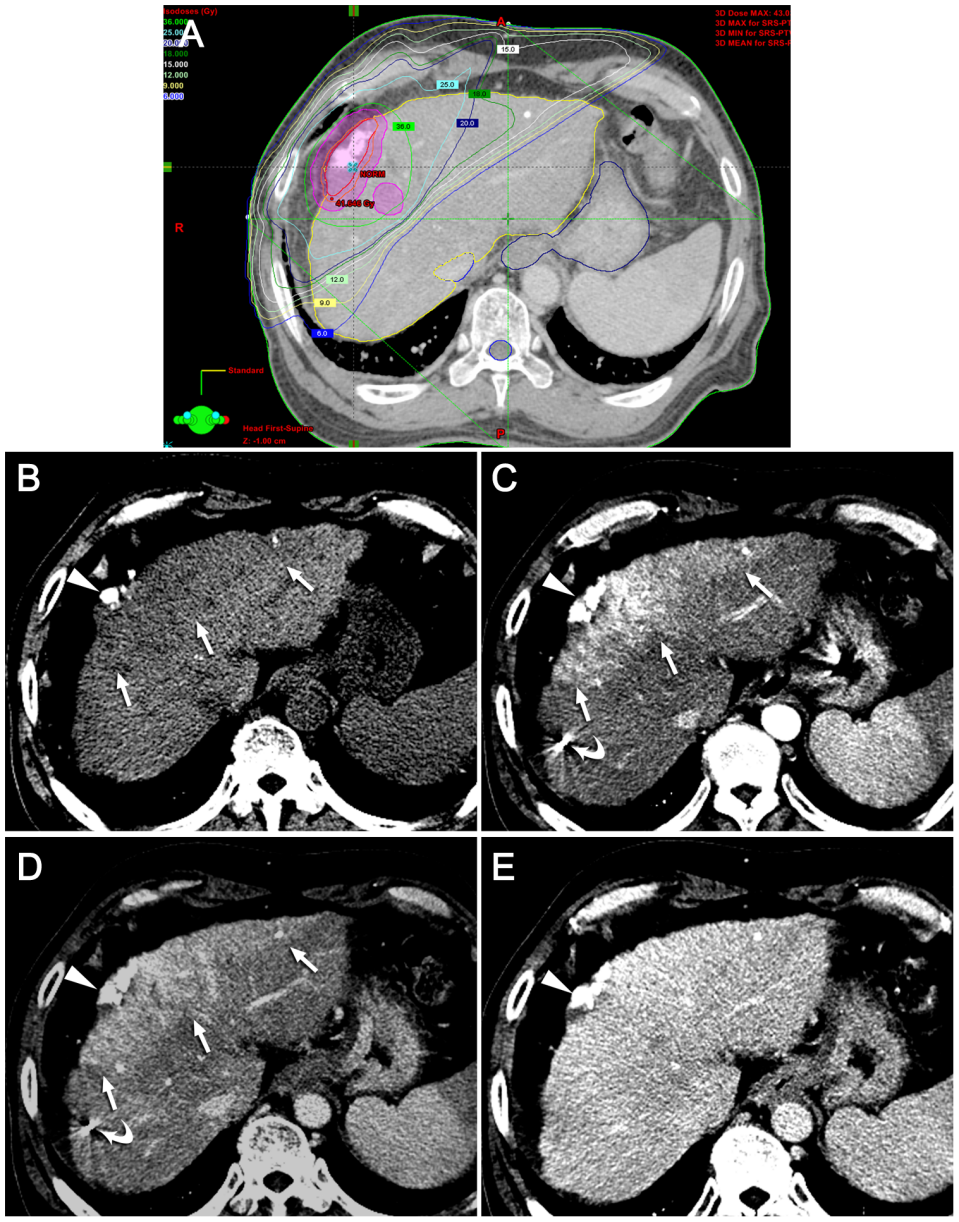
Typical hepatic attenuation difference seen on three months after SBRT. (A) Dose distribution is demonstrated on the isodose curve for SBRT. The planning target volume (PTV, purple circle) as well as the gross tumor volume (red circle) in the peripheral portion of the right lobe of the liver, was treated with 36 Gy of the total radiation dose. The irradiated hepatic parenchyma is delineated corresponding to the region inside the 15-Gy isodose line but outside the PTV boundary. (B-E) Multiphase CT images obtained three months after SBRT depict low attenuation (arrows) on noncontrast CT (B), high attenuation (arrows) on the arterial (C) and portal (D) phases, and isoattenuation on the delayed (E) phase, respectively. The target tumor (arrowheads) in the peripheral portion of the right lobe of the liver was treated using transarterial chemoembolization prior to SBRT. Fiducial markers are also seen (curved arrows).
